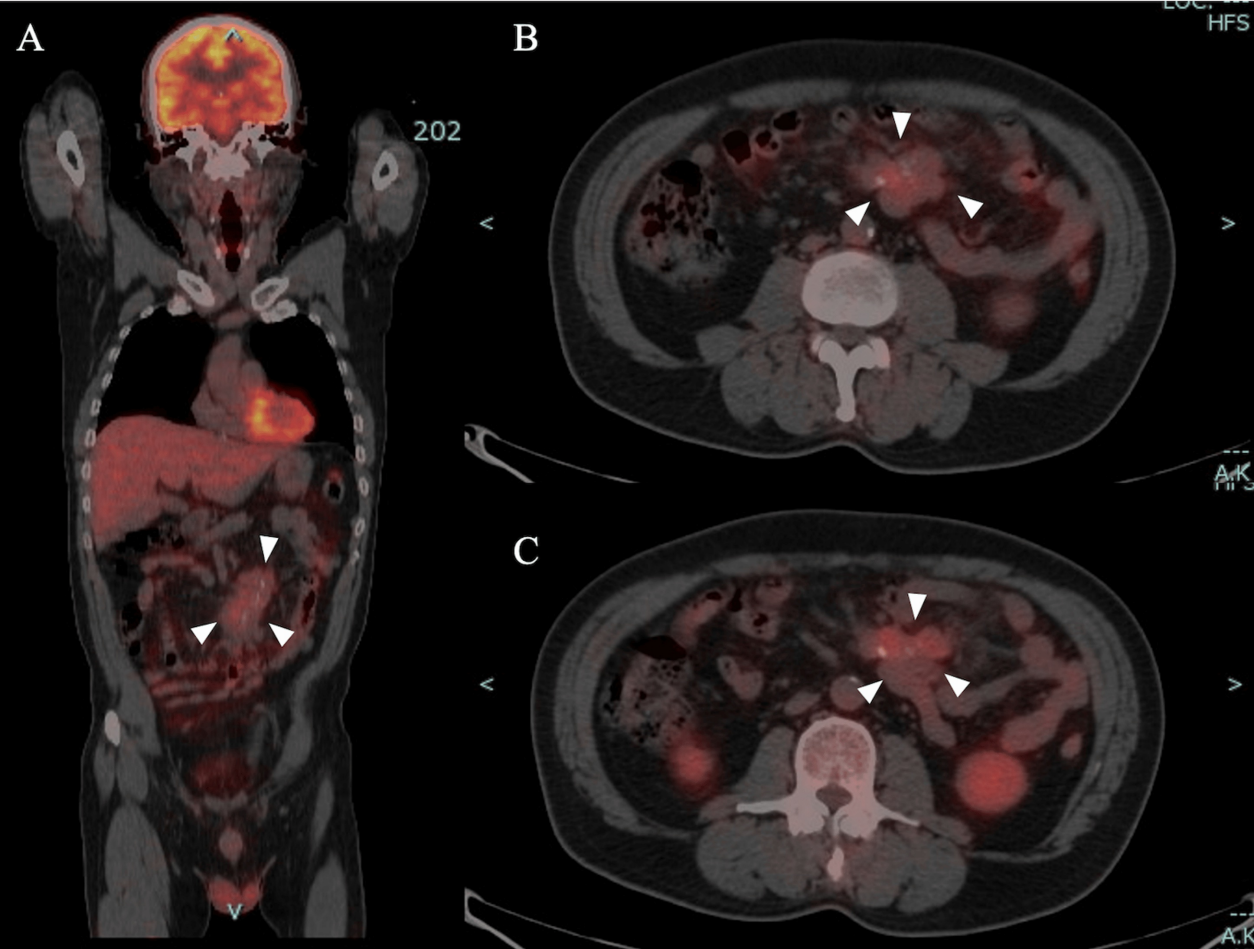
18F-Fluorodeoxyglucose positron emission tomography (FDG-PET)/CT. FDG-PET/CT identified FDG uptake in the mass (A, coronal section; B, horizontal section in the early phase), with a maximum standardized uptake value (SUV) of 3.11 in the late phase (C: delayed phase). CT: computed tomography
